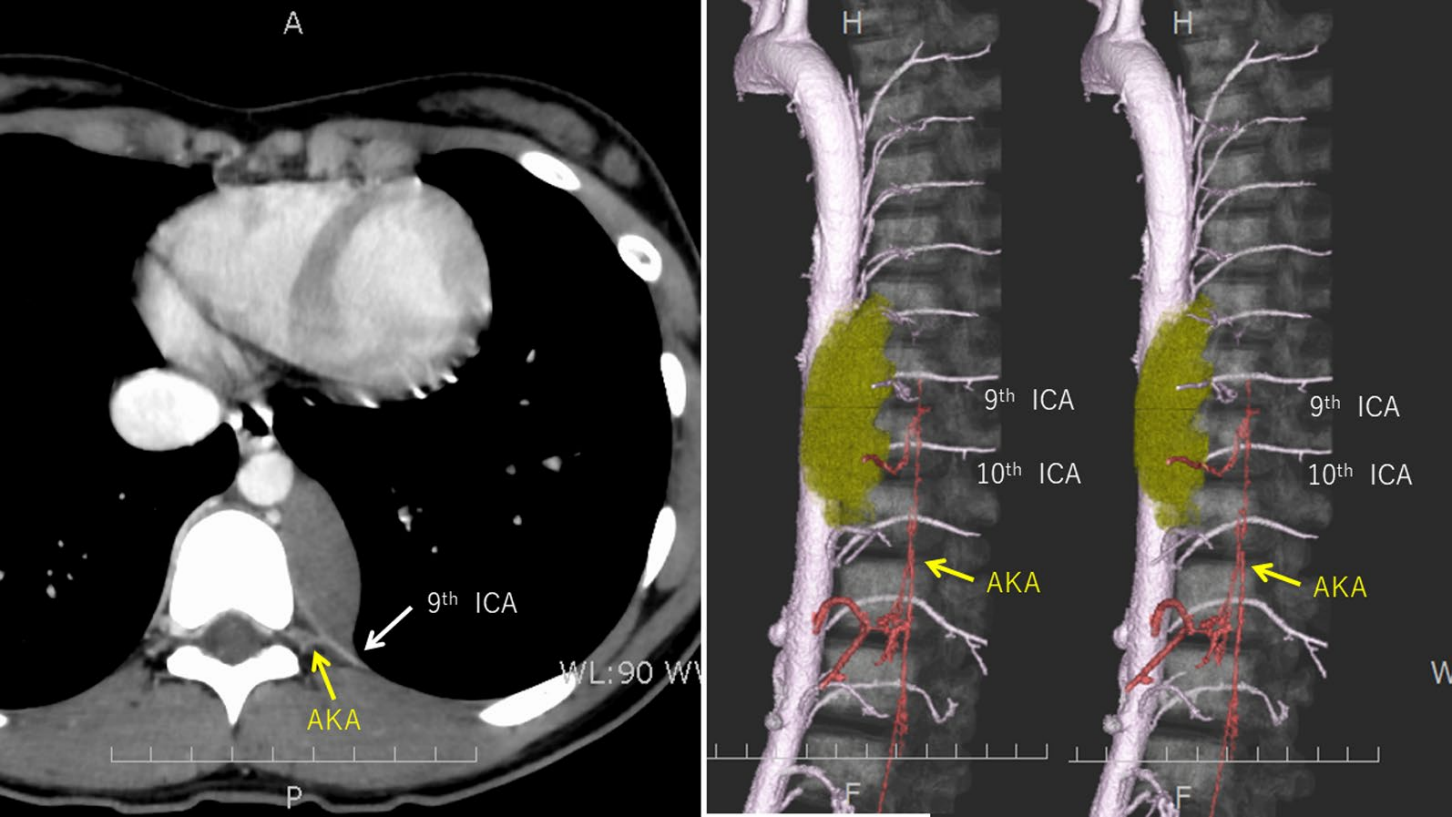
Contrast-enhanced computed tomography (CT) showed that the artery of Adamkiewicz arose from the 9th intercostal artery that ran between the tumor and the vertebrae. AKA, artery of Adamkiewicz; ICA, intercostal artery
